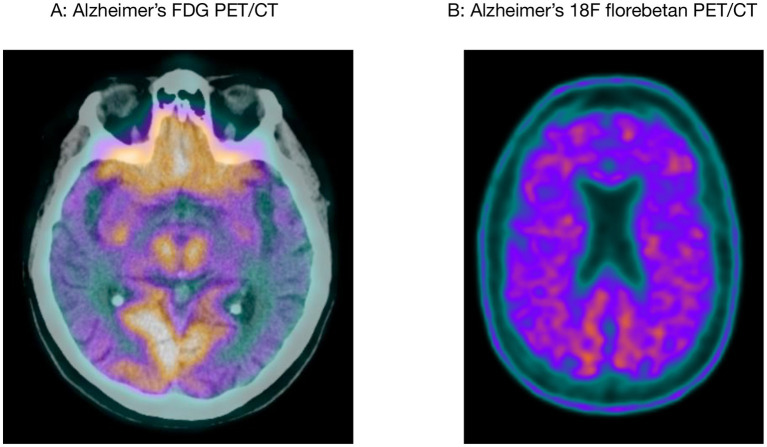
(A) Alzheimer’s FDG PET/CT. (B) Alzheimer’s ^18^F florebetan PET/CT.
